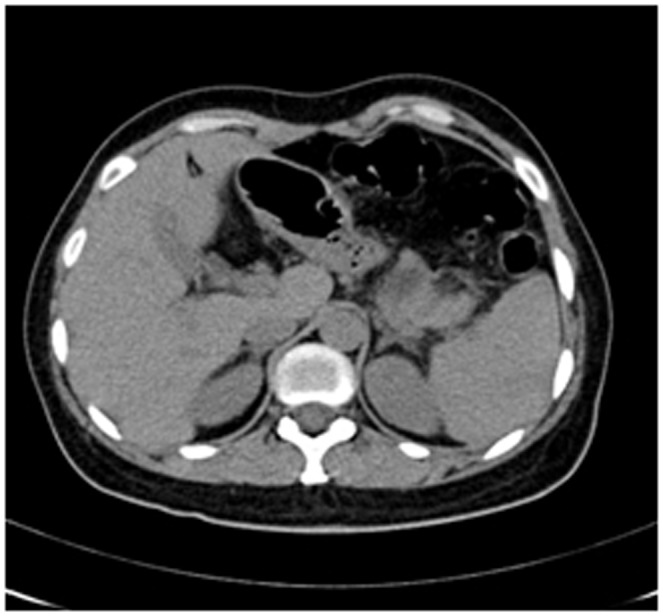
Enhanced CT showing peripancreatic narrow shadows.
